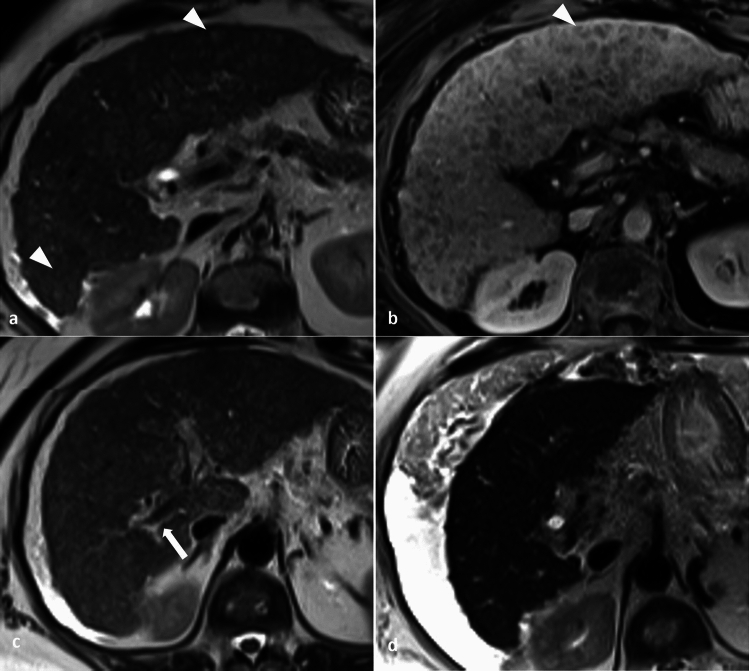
A 67-year-old female diagnosed with Primary Biliary Cholangitis at the age of 47. Axial T2 weighted MRI shows “periportal halo sign” as tiny, rounded areas of low signal intensity (arrowheads, a) and on postcontrast T1-weighted (arrowhead, b) around small peripheral portal vein branches distributed diffusely in both hemi-livers resulting in a markedly heterogeneous appearance. T2- weighted (arrow, c) shows periportal hyperintensity or “cuffing” around the right portal vein branch. MRI done at the age of 67 (image d) shows the absence of both periportal halo sign and periportal cuffing with the development of cirrhosis
